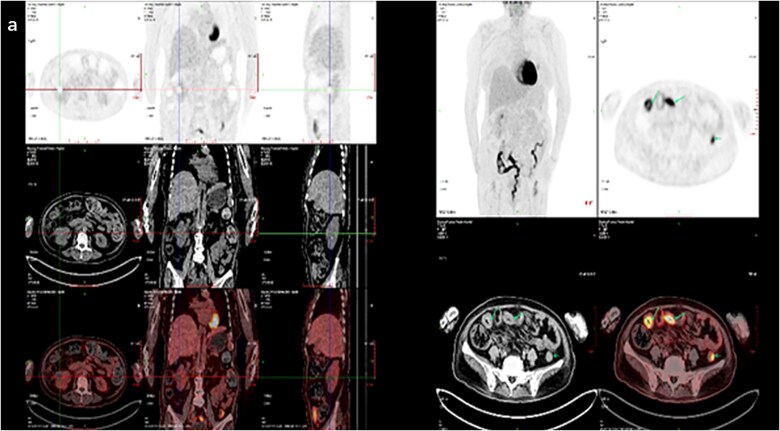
Follow-up positron emission tomography-computed tomography scan. (a) The tumour activity in the right kidney was obviously inhibited and the lesion was reduced.
